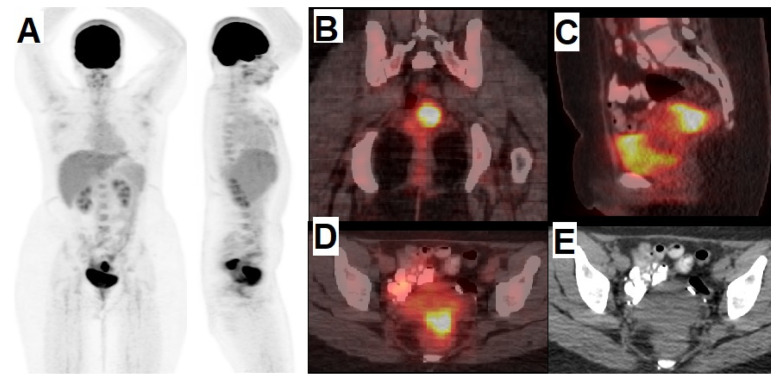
21-year-old female, known case of vaginal rhabdomyosarcoma, referred for staging. (A) anterior and right lateral MIP [^18^F]FDG PET/CT images. (B) coronal, (C) sagittal, (D) transvers pelvis fused [^18^F]FDG PET/CT images and (E) corresponding transvers pelvis CT image showed hypermetabolic primary tumor without evidence of regional lymphadenopathy or distance metastasis.
